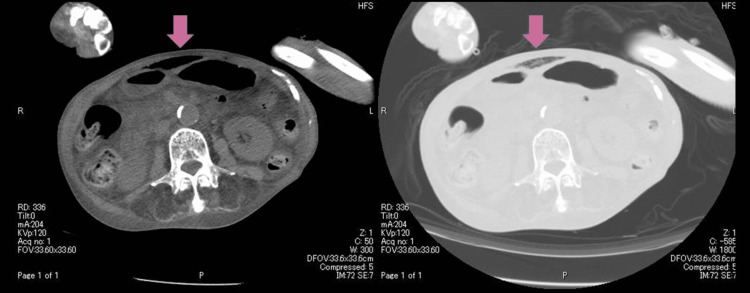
Abdominal wall emphysema on admission In the left image, it is difficult to differentiate between abdominal wall emphysema and intra-abdominal free air. Changing the CT window width as shown in the image on the right reveals that it is abdominal wall emphysema
